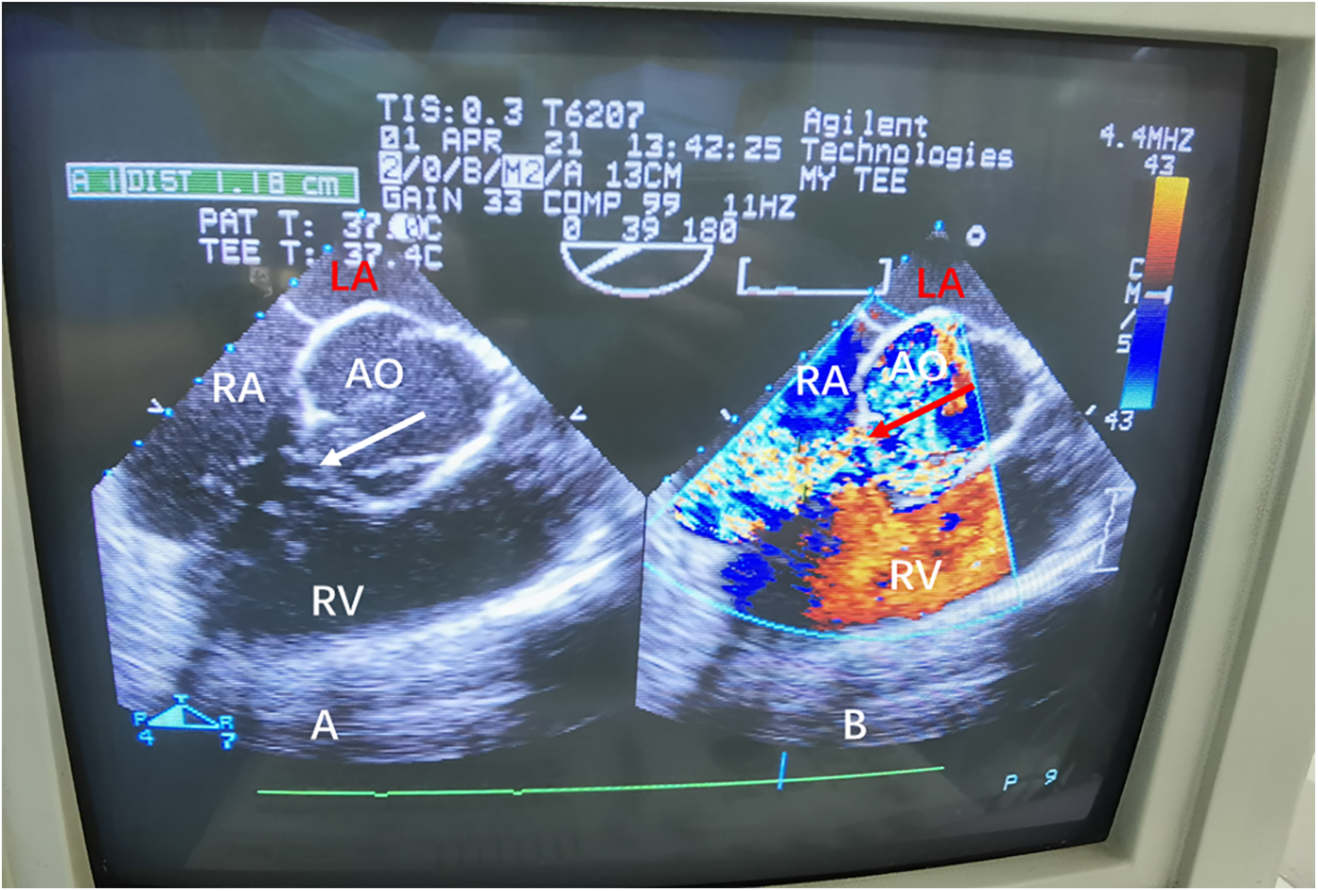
(A) Transesophageal echocardiography demonstrating the characteristic “windsock appearance” of the sinus of Valsalva aneurysm. (B) Color Doppler flow demonstrating the large left-to-right shunt (arrow) from the ruptured sinus of Valsalva aneurysm into the right atrium. Ao, aorta; LA, left atrium; RA, right atrium; RV, right ventricle.
